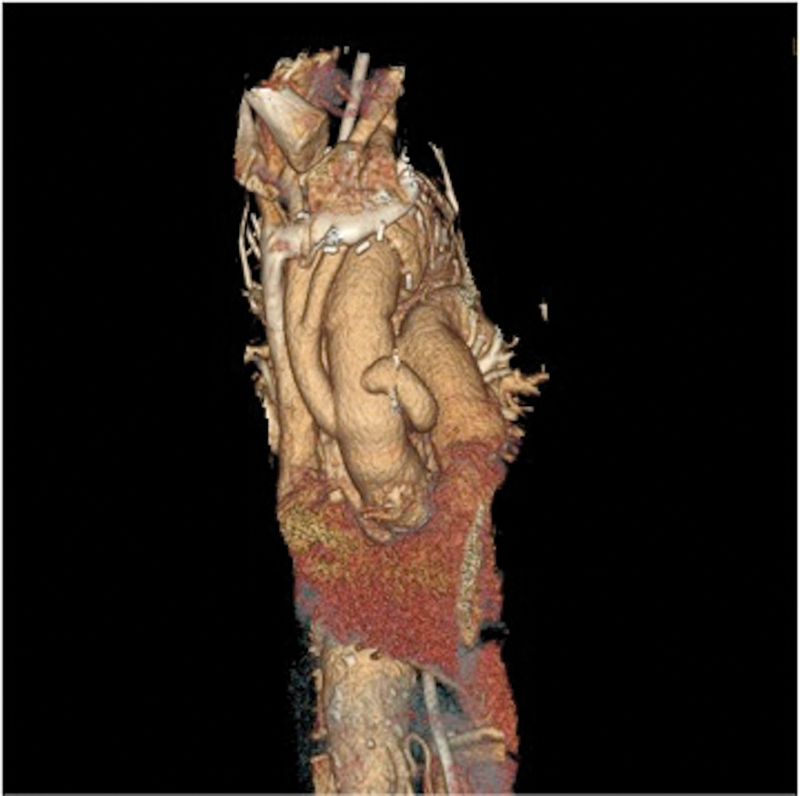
Computed tomography—three-dimensional reconstruction image: The 10 mm prosthetic graft on this occasion is routed to the left side of the tube graft due to the presence of the trifurcated graft.
